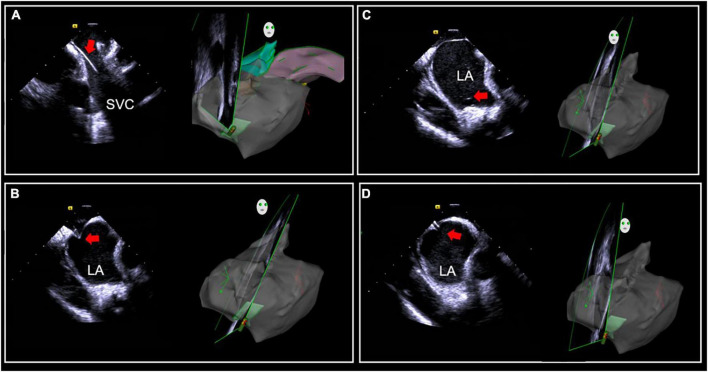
Intracardiac (ICE)-guided zero X-ray transseptal puncture. (A) Guidewire and sheath seen in SVC. (B) “Tenting sign” in the fossa ovalis by puncture needle sheath. (C) Saline injection microbubbles into the left atrium after the needle is inserted. (D) Needle tip into the left atrium. SVC, superior vena cava; LA, left atrium.
